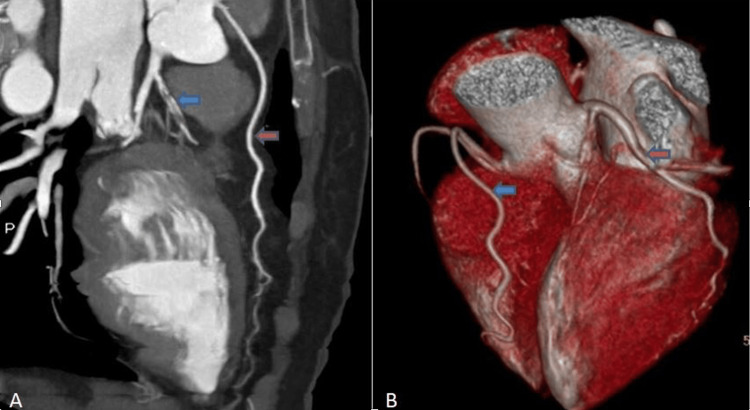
(A) Reformatted CTCA and (B) volume rendering technique images, showing thrombosed LAD (blue arrows) and accessory LAD (red arrows) CTCA: computed tomography coronary angiography; LAD: left anterior descending artery
